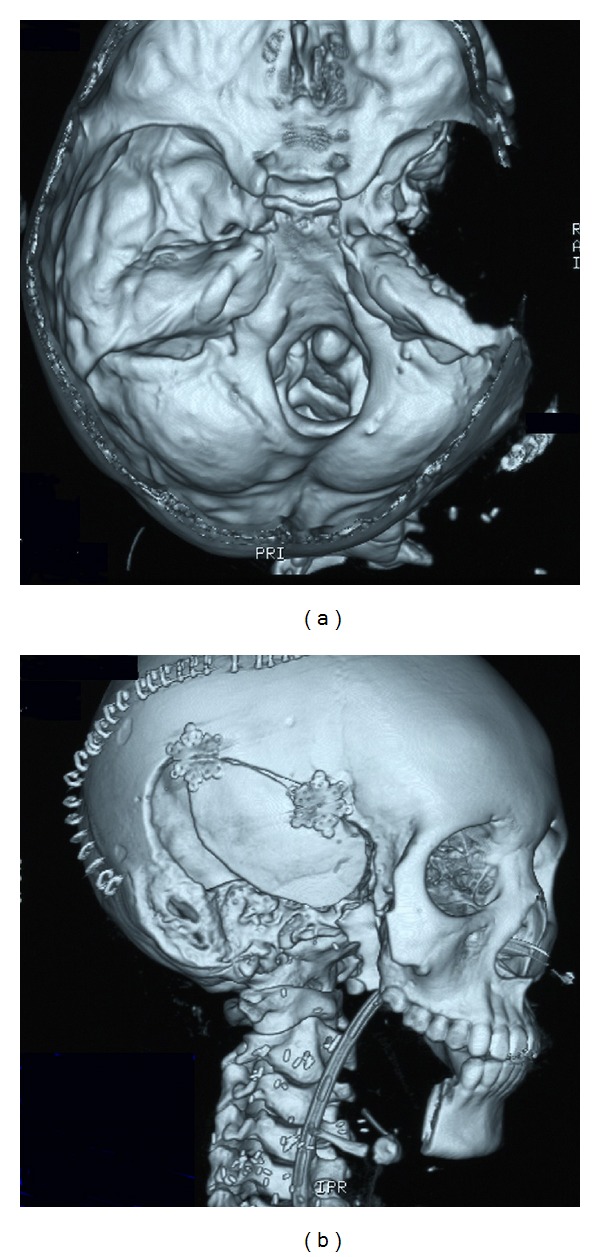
Postoperative 3D CT. The temporomandibular joint capsule, external auditory meatus, and mandibular dissection were removed. The inner ear was retained.
